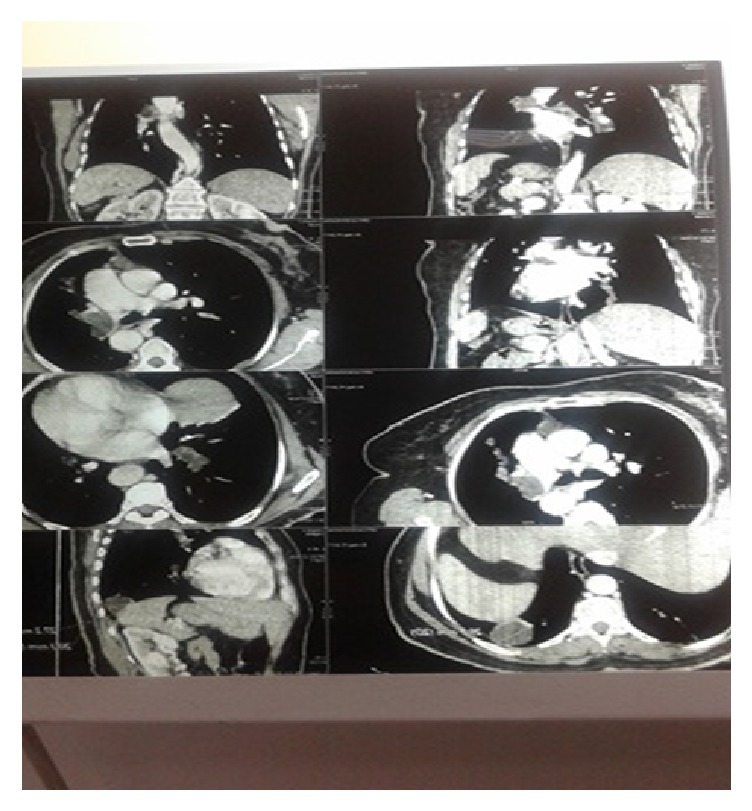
CT chest scan with a 31 cm dilation of the pulmonary trunk with a partial occlusion of the left pulmonary artery and a complete occlusion of the right pulmonary artery by a fluid attenuation lesion and a subpleural cyst of the inferior lungular segment measuring 19 mm.
